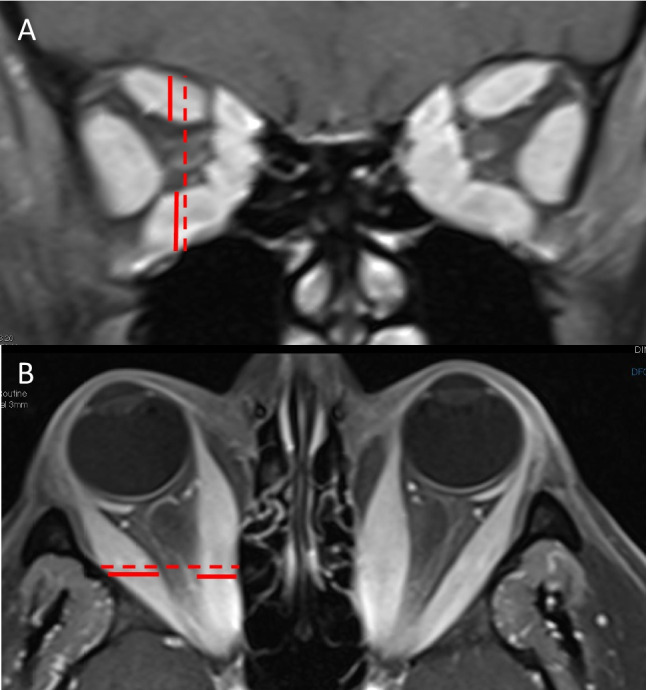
Right dysthyroid optic neuropathy. Mid-coronal fat-suppressed contrast-enhanced T1-weighted MRI (A) showing the vertical muscle index, which is a ratio of the sum of the vertical height of the superior and inferior recti (solid line) to the vertical orbital height (dashed line). Axial fat-suppressed contrast-enhanced T1-weighted MRI (B) showing the horizontal muscle index, which is a ratio of the sum of the diameters of the medial and lateral recti (solid line) to the horizontal orbital distance (dashed line)
